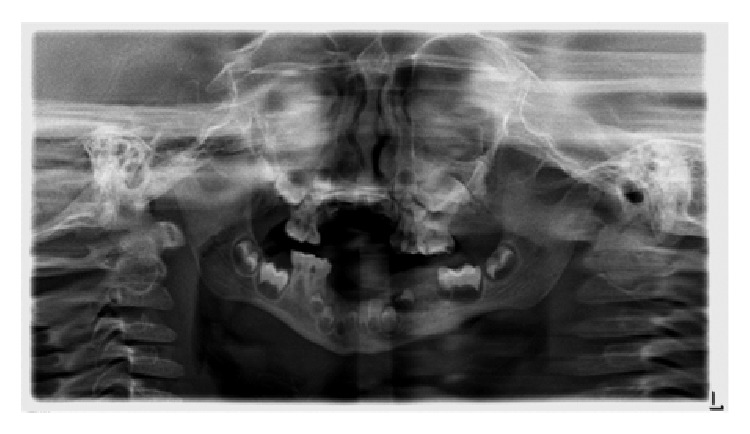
An orthopantomogram (OPG) taken at age of four, showing the maxilla and mandible with few standing deciduous teeth and maturation stage of permanent maxillary and mandibular teeth.
